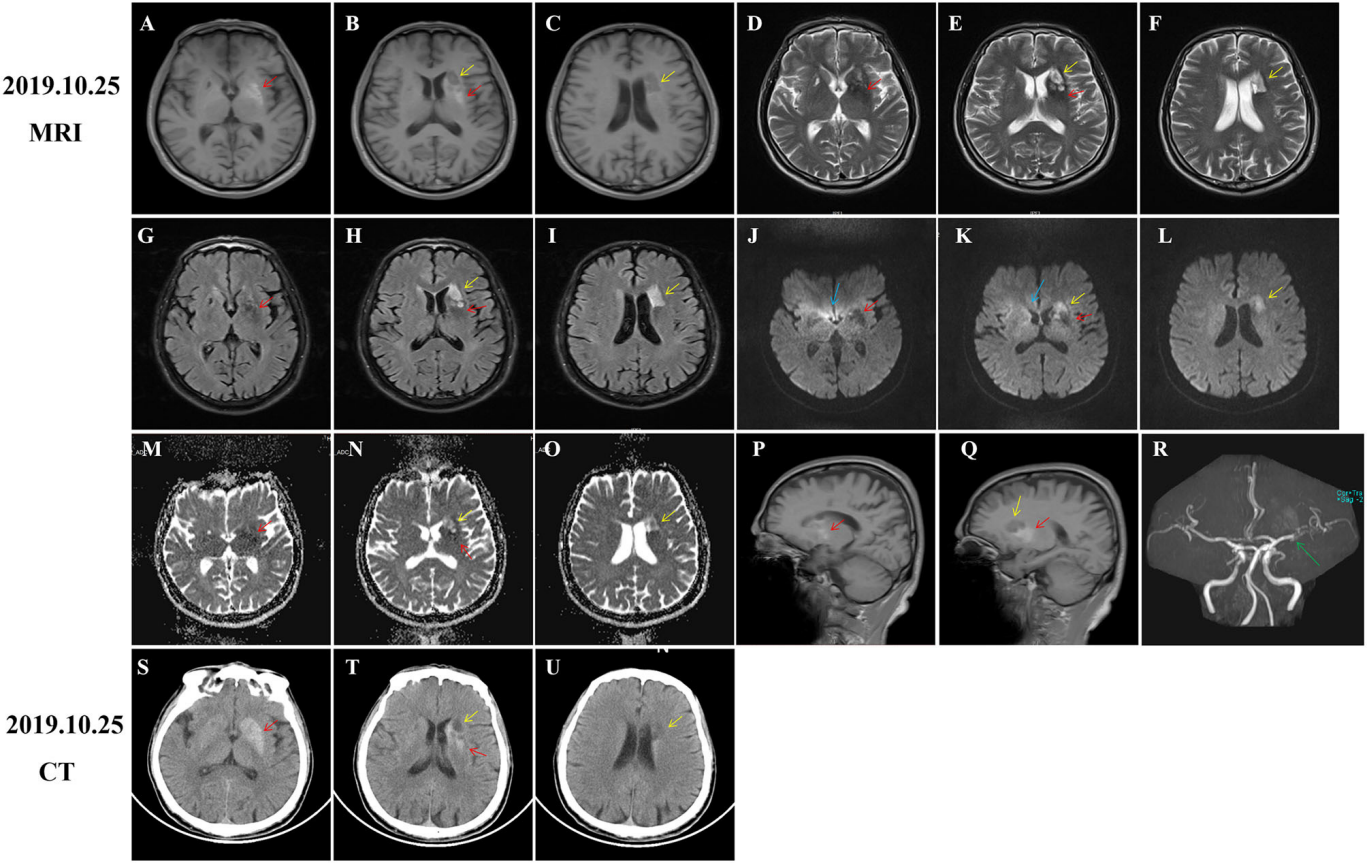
Computed tomography (CT) and MRI of brain at admission. Diabetic striatopathy (DS)-associated striatal abnormalities were marked by red arrows, while infarction focuses were marked by yellow arrows. Typical imaging manifestations of DS were found in the left striatum with T1 hyperintensity [(A,B), coronal images; (P,Q), sagittal images] on MRI and hyper-density on CT images (S,T). The hypointensity on T2 (D,E), fluid-attenuation inversion recovery (FLAIR) (G,H), and apparent diffusion coefficient (ADC) (M,N) images, as well as equal signal intensity on DWI images (J,K) were also observed in DS-associated striatal lesions. Subacute cerebral infarctions showed T1 hypointensity [(B,C) coronal images; (Q) sagittal image], T2 hyperintensity (E,F), FLAIR hyperintensity (H,I), DWI hyperintensity (K,L), and slight ADC hyperintensity (N,O) on MRI images, as well as hypo-density on CT images (T,U). MRA images disclosed obvious stenosis of the left middle cerebral artery [(R), green arrow]. There were several artifacts on ADC images [(J,K), blue arrows].
